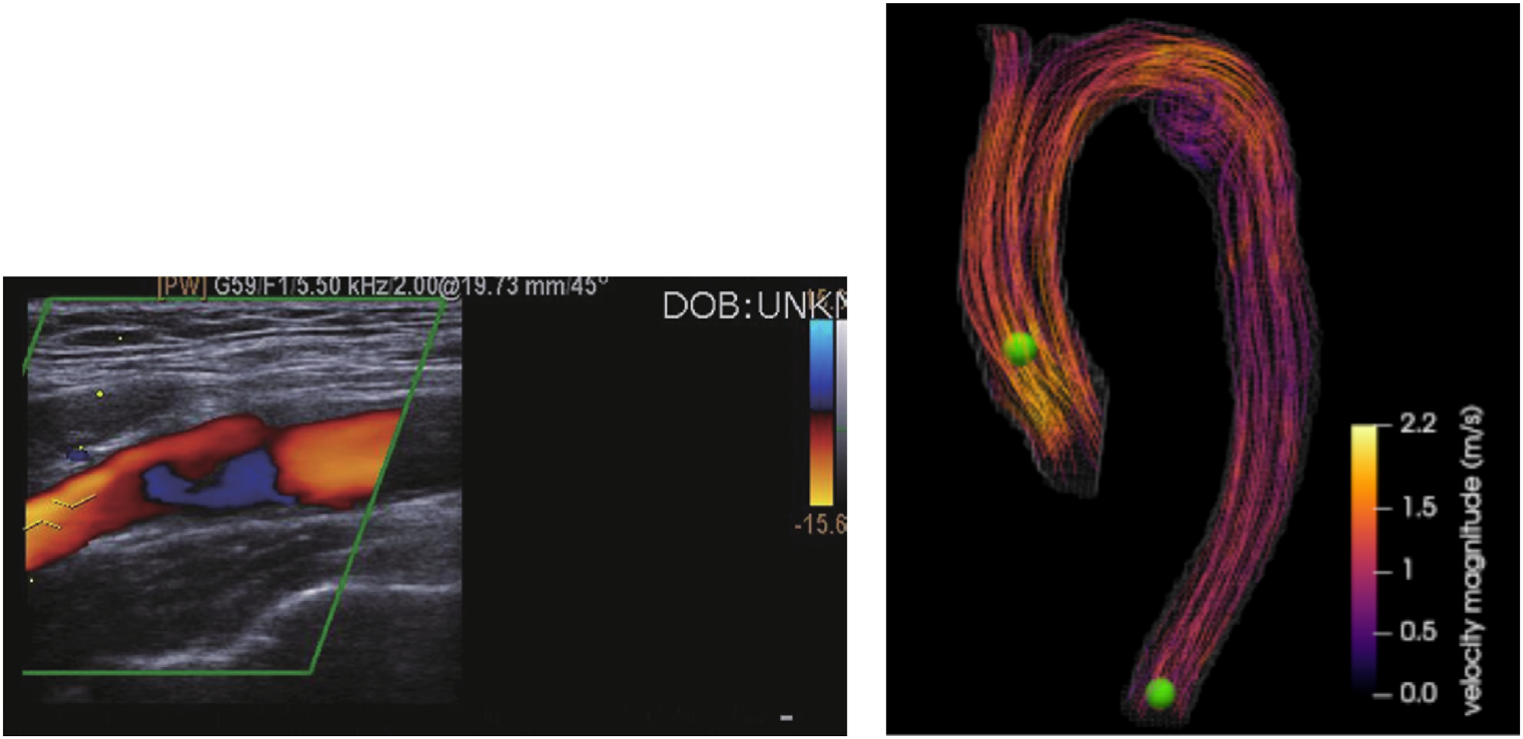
Blood flow velocity measurements. Doppler measurement of the internal carotid artery^45^ (left) and 4D Flow MRI of an CoA^41^ (right). Reprinted with permission
